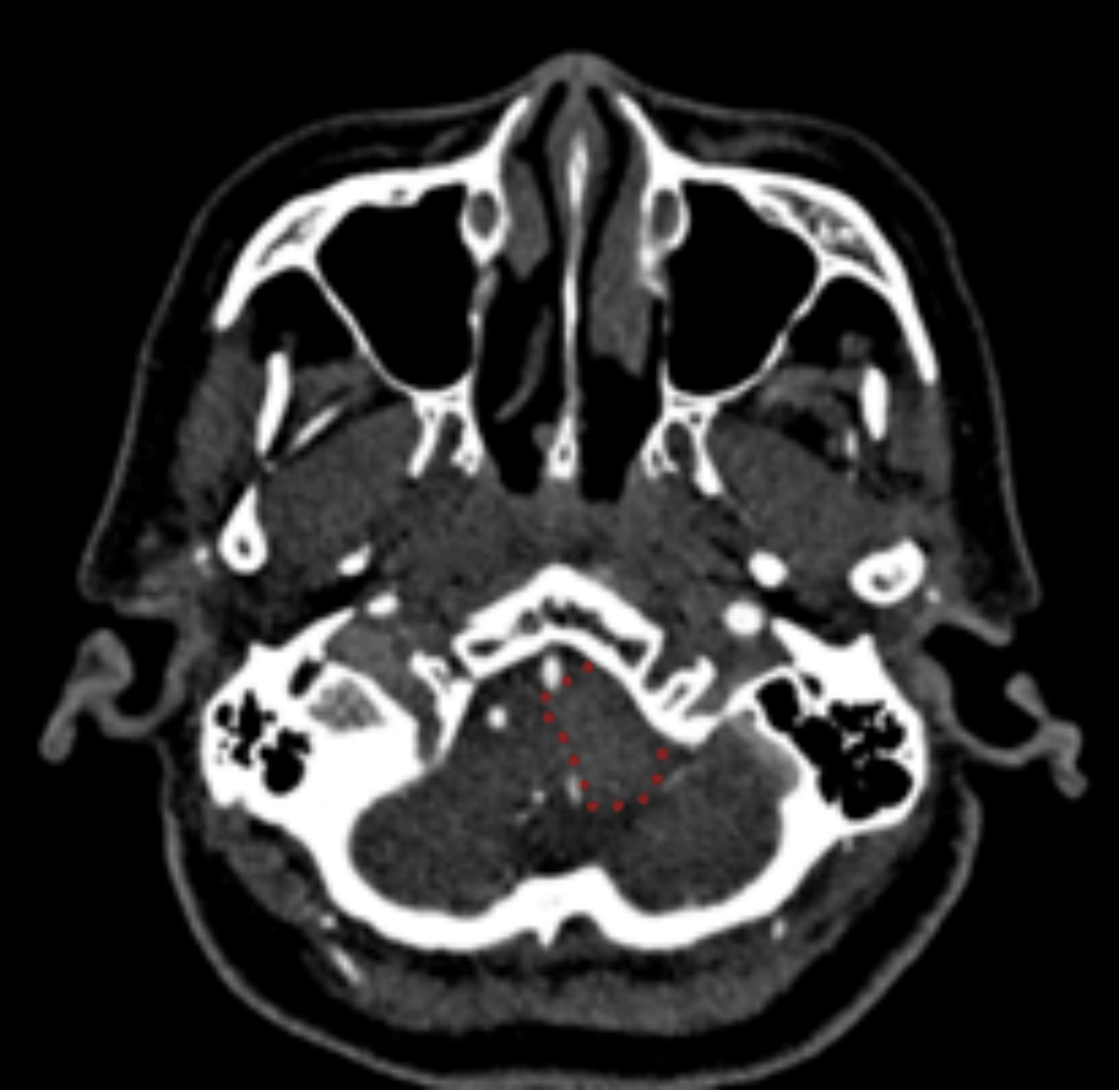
Contrast-enhanced CT of the brain showing an isodense lesion with minimal contrast uptake in the foramen magnum, adjacent to the left vertebral artery. The lesion boundary is outlined with red dots.
